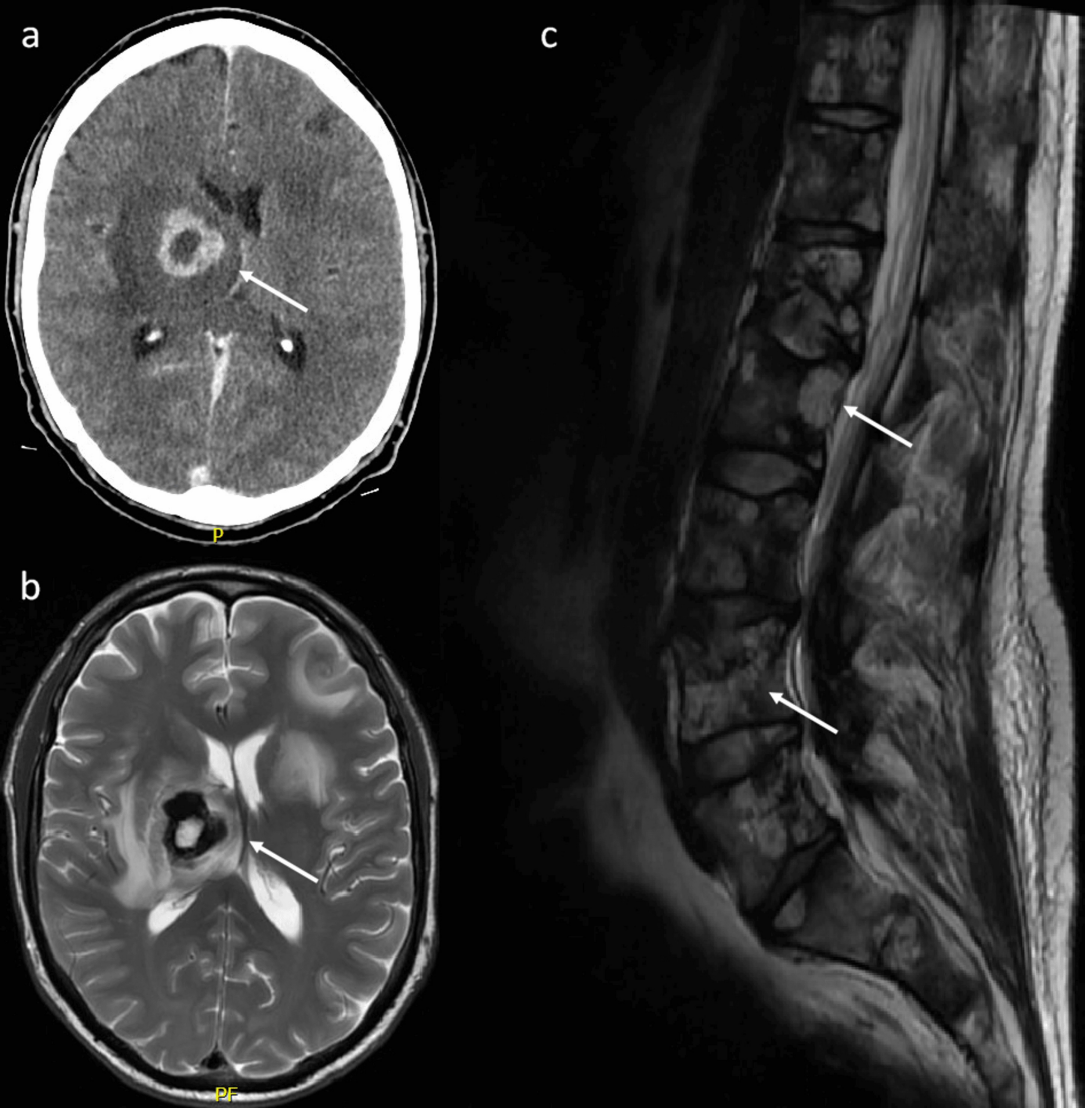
CT brain (a) and MRI brain (b) identifying intracranial metastases, and MRI lumbar spine (c) showing osseous metastatic disease, highlighted by arrows
